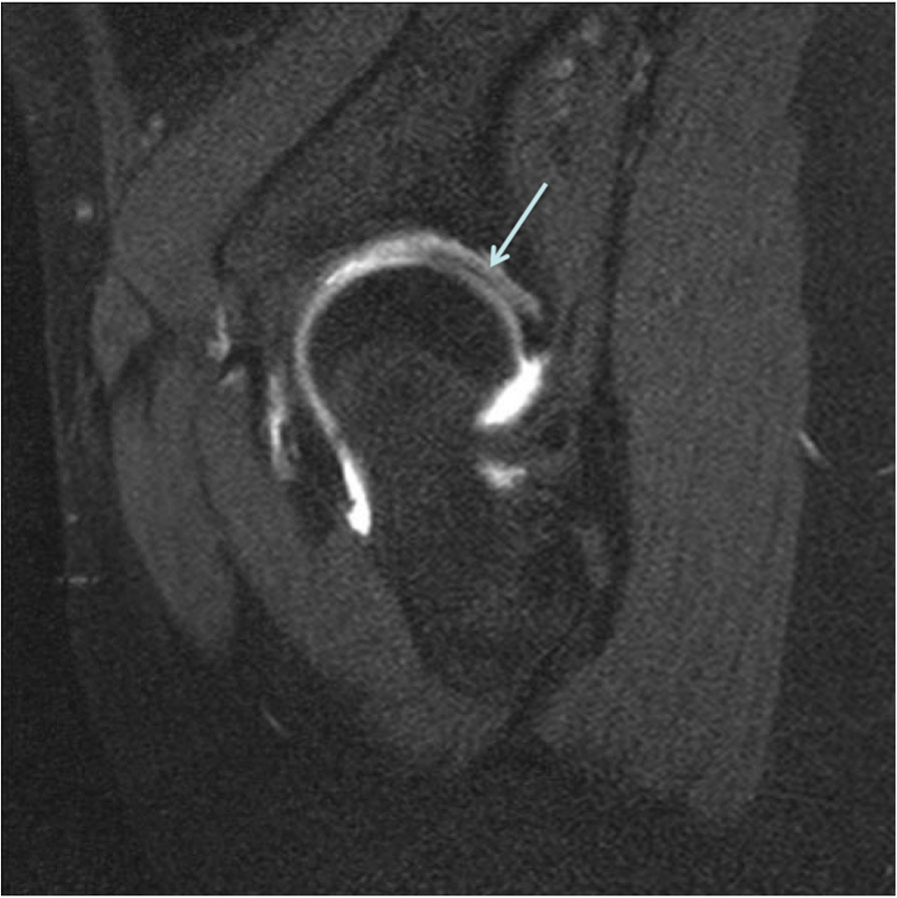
Direct magnetic resonance arthrography of a 31-year-old woman with left developmental dysplasia of the hip. The T1WI MRI image reveals a labral inversion, shown as a stripe of low signal between articular cartilages indicated with an arrow
